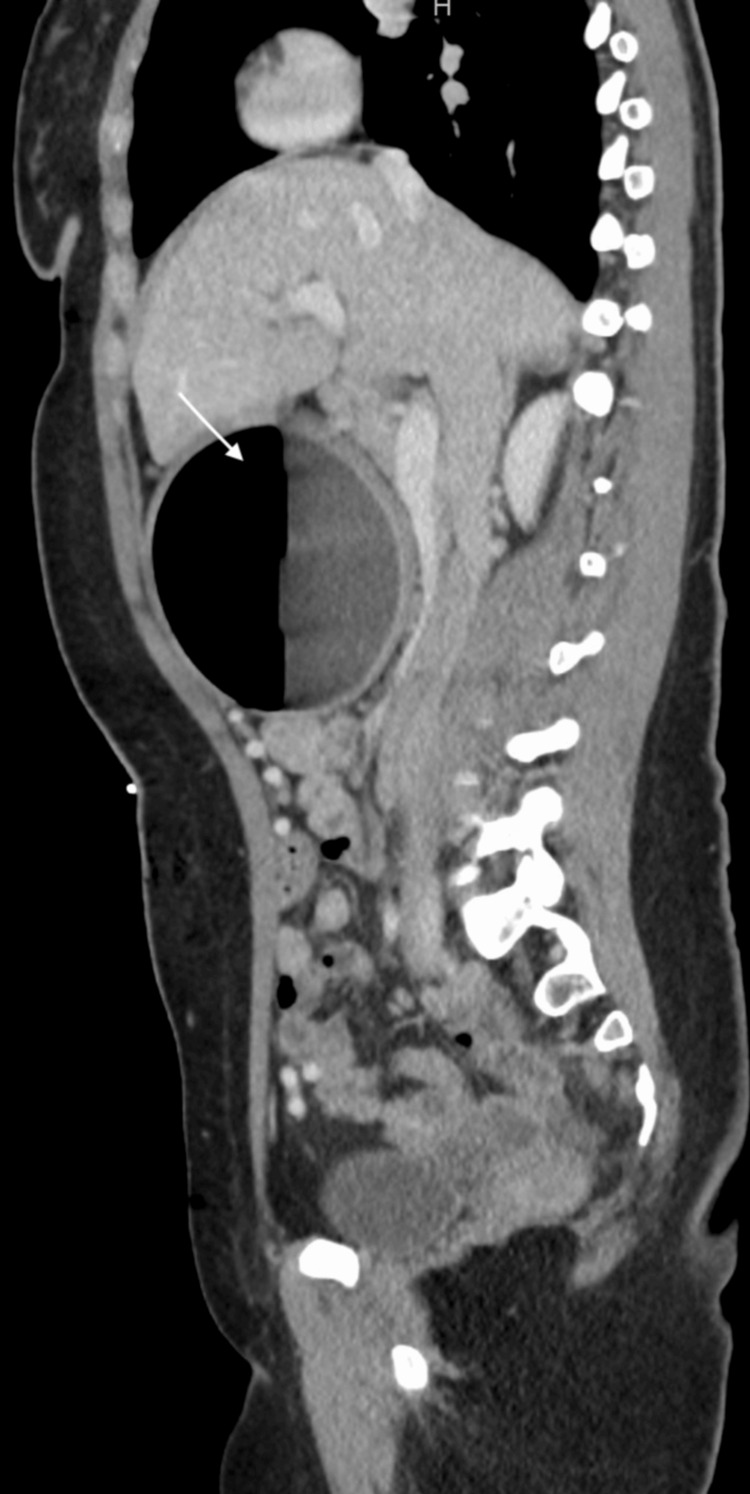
Sagittal view of CT scan of patient’s abdomen with the intragastric balloon from referral hospital
